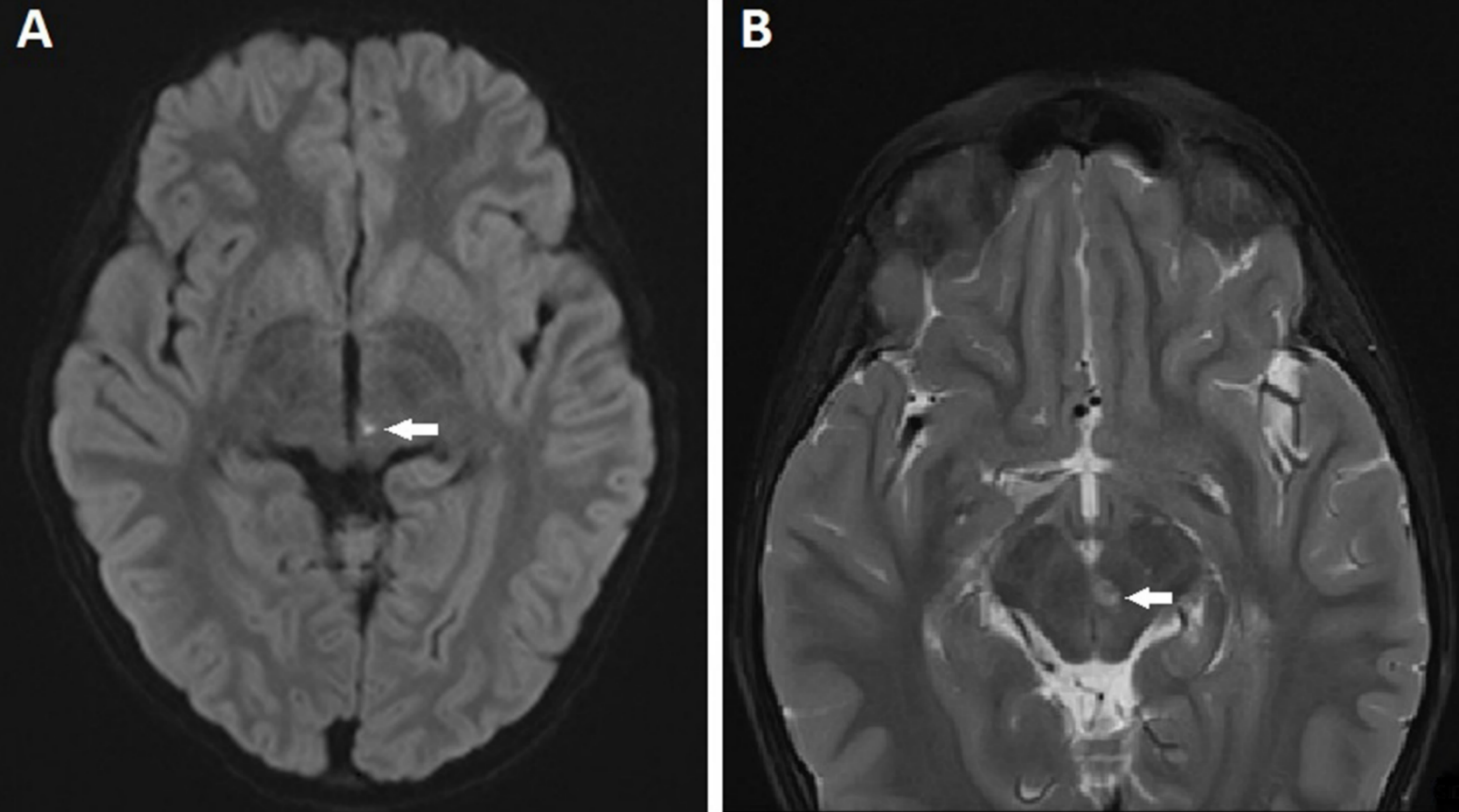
MRI scan of the brain showing tiny infarct (white arrow) in the left paramedian rostral upper midbrain at the level of the red nucleus; (A) axial diffusion weighted image showing restricted diffusion in the T2 hyperintensity, and (B) axial T2 fat saturated image showing T2 hyperintense focus
